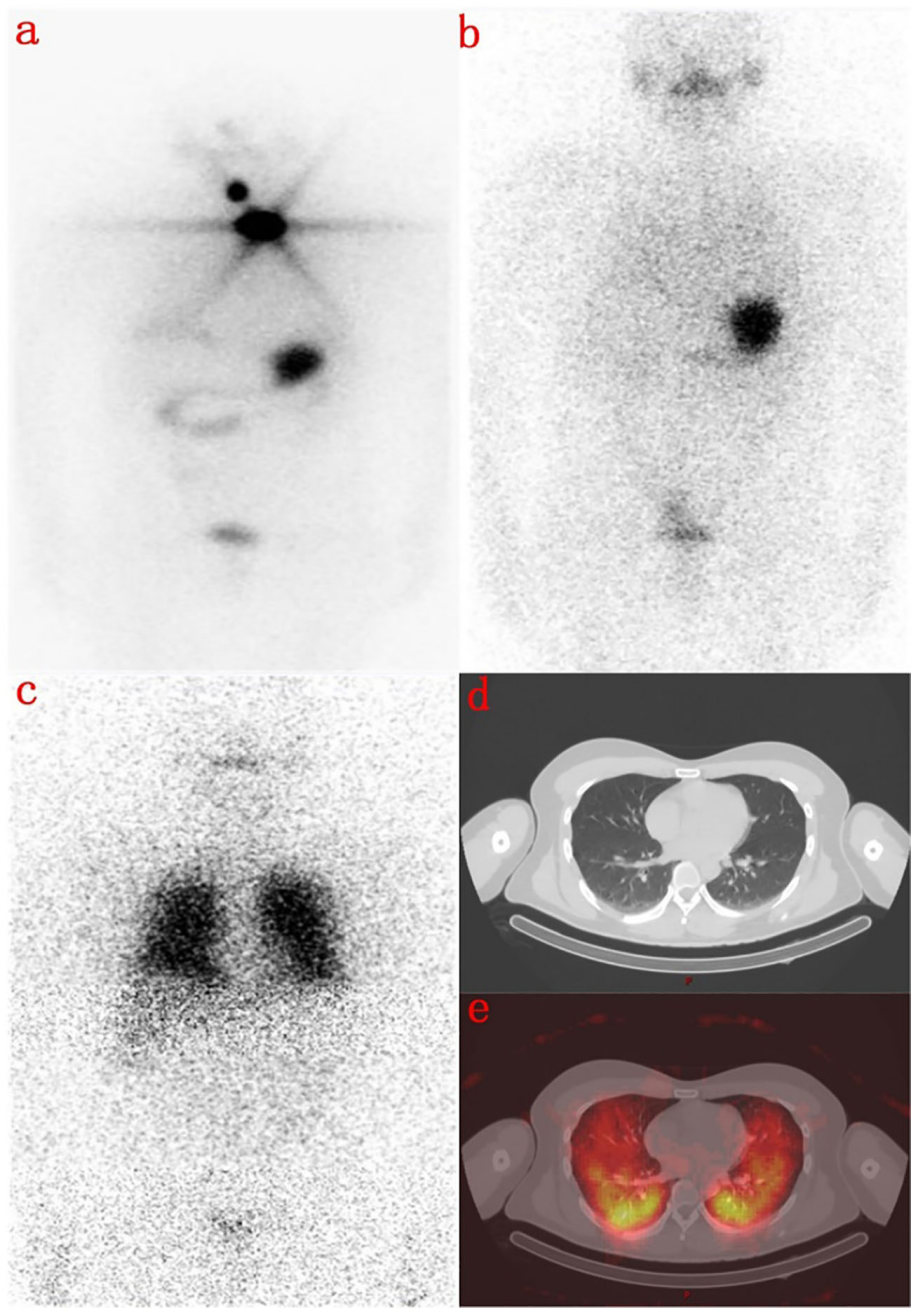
A 31-year-old male with total thyroidectomy one year ago. (A) shows a radioactive concentration in the residual thyroid on the first ^131^I WBS imaging performed 8 months ago. (B) shows that there was no abnormal concentration of radioactivity on the day 3 imaging after second ^131^I therapy. (C) shows that there was no display in the residual thyroid but a diffuse abnormal radioactive concentration in both lungs in the day 7 imaging. (D) is the Chest CT image, and (E) shows that lung metastasis of thyroid cancer on the day 7 with SPECT/CT fusion image.
